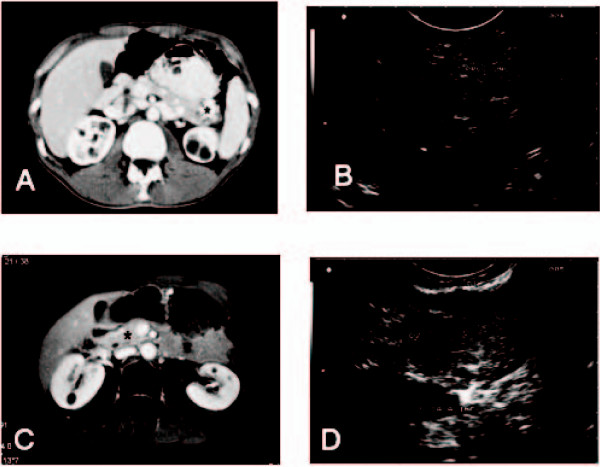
Imaging studies before initial and re-operation. (A) Pre-operative computed tomography scan before the initial operation. (B) Pre-operative endoscopic ultrasound before the initial operation (asterisk indicates the tumor; Proc. unc., normal appearing uncinate process). (C) Pre-operative computed tomography scan before the re-operation. (D) Pre-operative endoscopic ultrasound before the re-operation.
